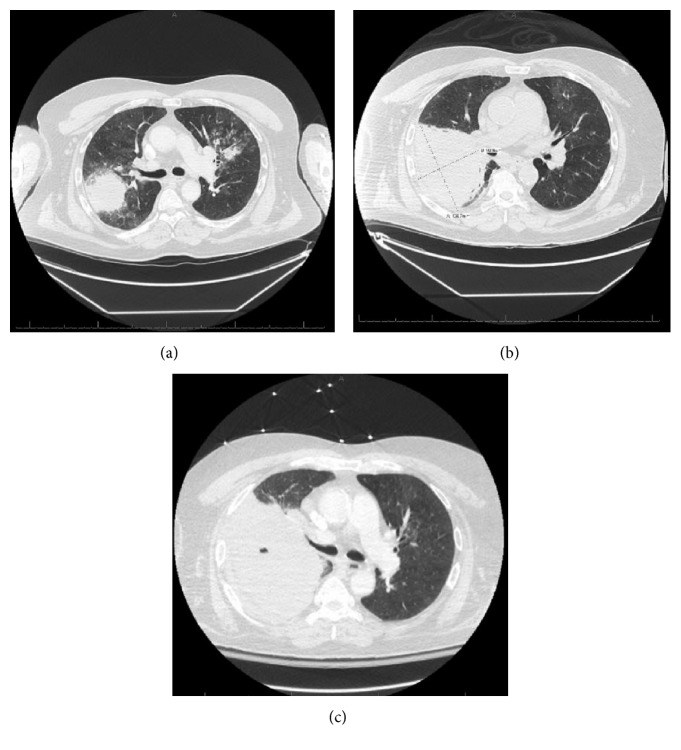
CT chest from day of admission (a), day of fine needle biopsy (b), and prior to VATS (c).
